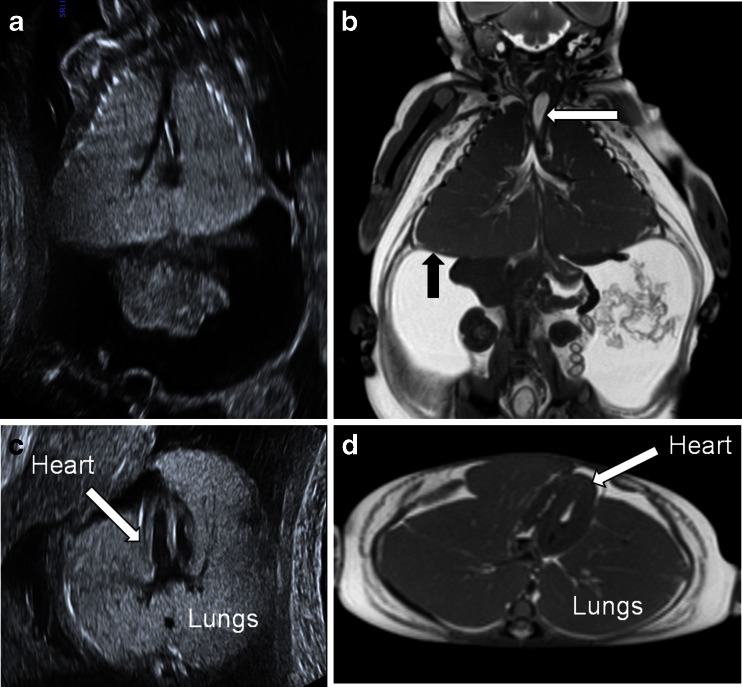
Case 1. Imaging in a male foetus (same as in Fig. 1) with congenital high airway obstruction syndrome (CHAOS), including antenatal US at 20 weeks’ gestation (a, c) and postmortem coronal (b) and axial (d) high-resolution T2-weighted postmortem MRI following termination at 24 weeks’ gestation. Postmortem MRI shows disproportionately gross ascites (relative to subcutaneous oedema) on coronal (b) and axial (d) abdominal imaging, marked distension of both lungs with eversion of the diaphragm and a centrally placed heart, typical features of CHAOS. The heart and lungs are indicated on axial MR image (d). Coronal MR image (b) demonstrates the fluid-filled airway (*white arrow*) below the level of the obstruction, as well as the eventration of the diaphragm (*black arrow*)
